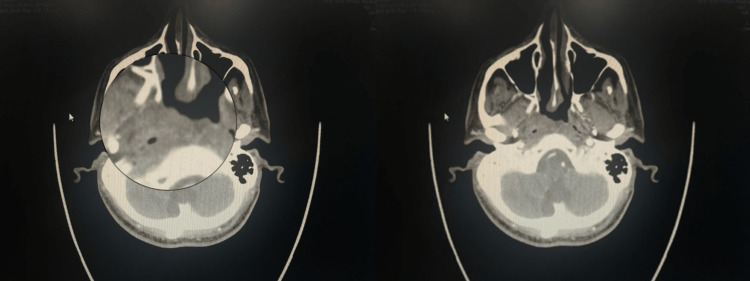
Axial computed tomography (CT) scan of the neck showing prominent asymmetry of the nasopharyngeal soft tissue, more on the right side, with no CT features suggestive of a retropharyngeal abscess.
